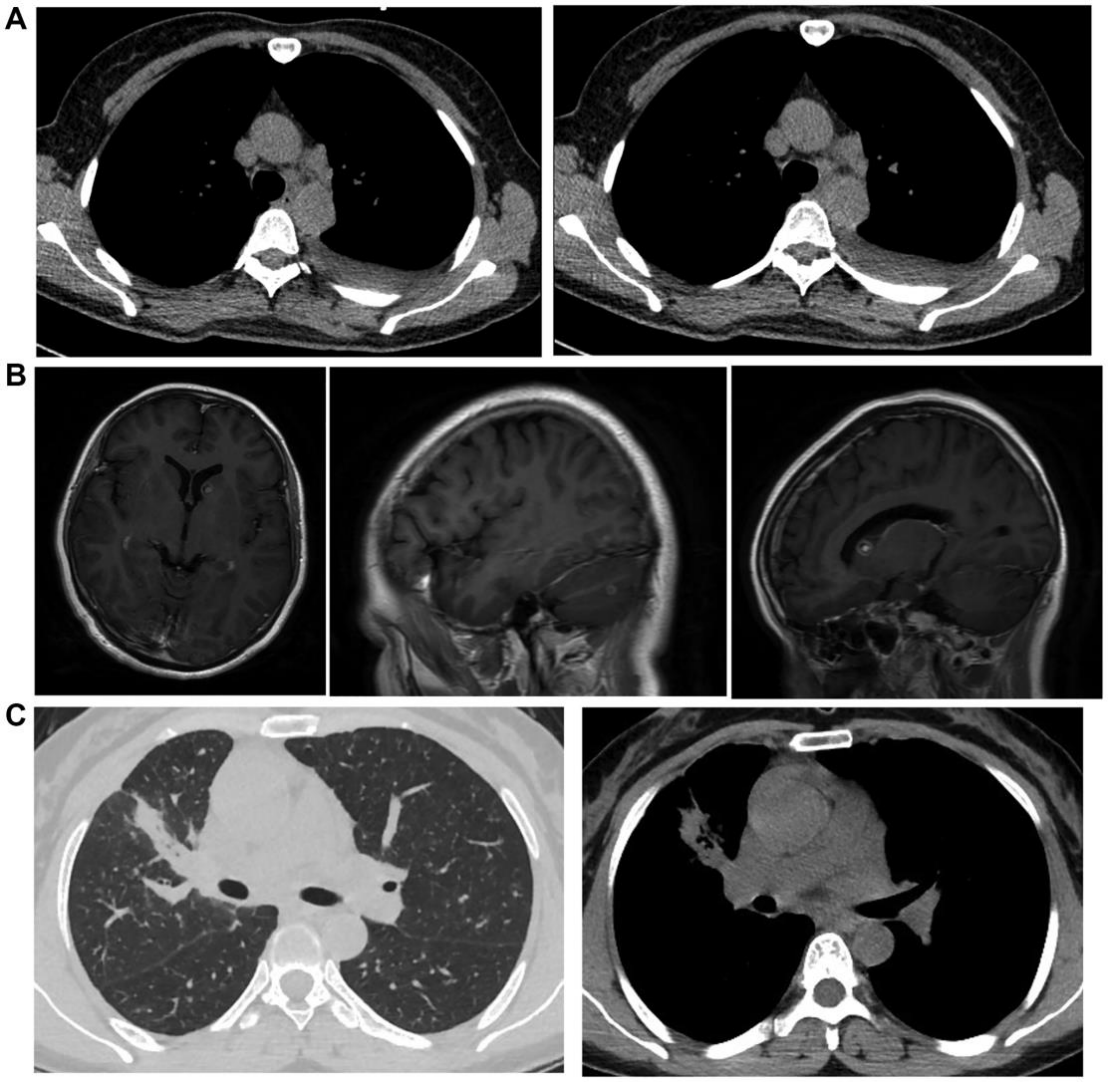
The MRI images of metastatic lung cancer patients. (A) MRI image of mediastinal lymph node metastasis and left side pleural effusion. (B) MRI image of brain metastatic non-small cell lung cancer. (C) MRI image of non-small cell lung cancer in the upper lobe of the right lung with metastasis in both lungs and mediastinum.
